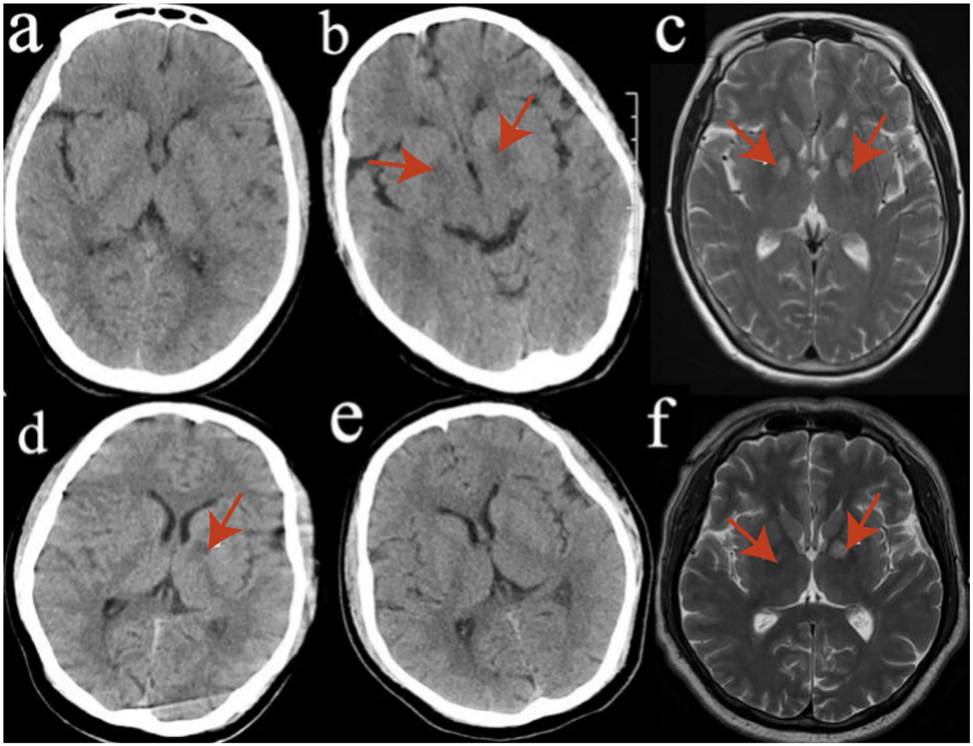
Representative cranial imaging of two patients. Note: (a) Day 1 cranial CT of case 2 showing no obvious abnormalities. (b) Day 6 cranial CT of Case 2 displaying symmetrical low-density changes in the bilateral globus pallidus (red arrows). (c) Day 2 cranial MRI of Case 2 demonstrating symmetrical hyperintensities in the bilateral globus pallidus (red arrows). (d) Day 1 cranial CT of Case 4 showing a low-density lesion in the left globus pallidus (red arrow). (e) Follow-up CT scan at 2 weeks in Case 4 showing resolution of the lesion. (f) Day 2 cranial MRI of Case 4 demonstrating bilateral globus pallidus hyperintensities (red arrows). CT: computed tomography; MRI: Magnetic resonance imaging.
